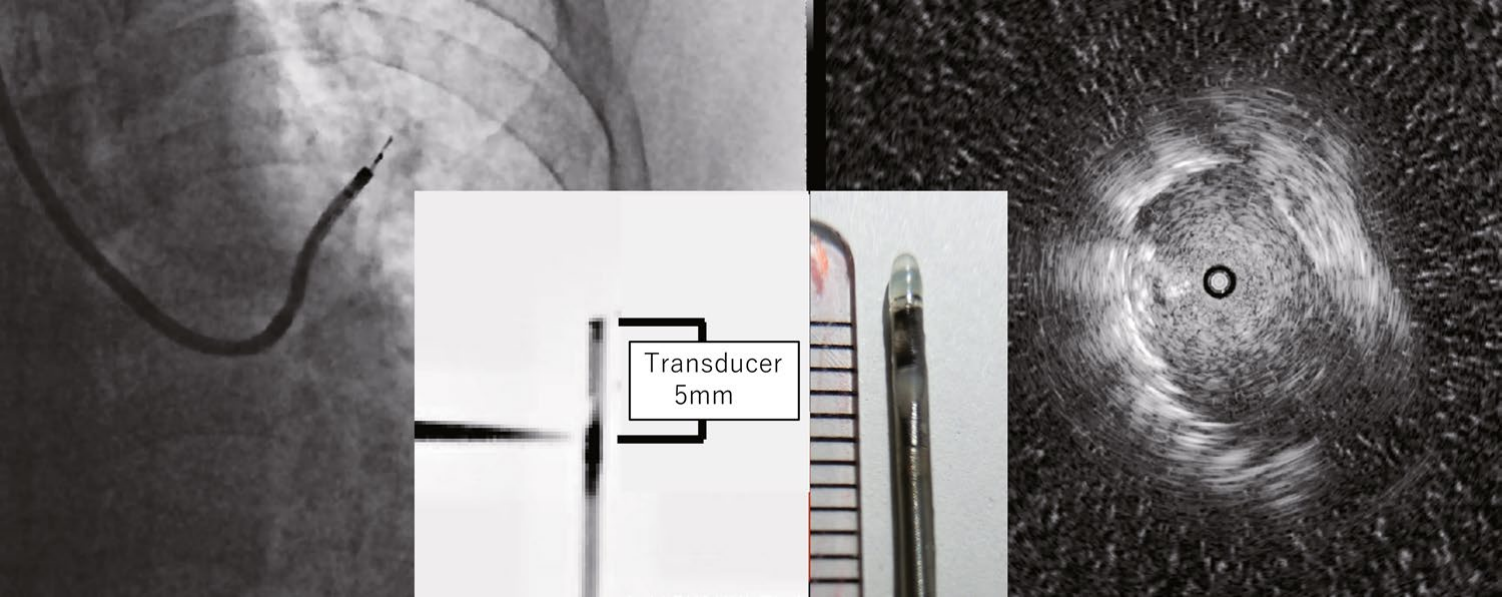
Criteria for CL-TBB. The distance from the RP-EBUS probe tip to the ultrasound transducer is 5 mm. “CL-TBB” was considered when the distance from the bronchoscope tip to the lesion was ≤ 10 mm under fluoroscopy and RP-EBUS images.
